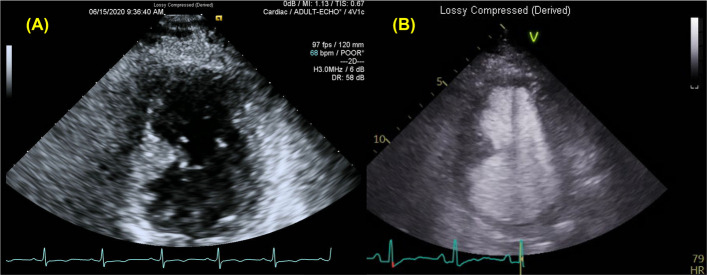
Contrast echocardiography. A Transthoracic echocardiography revealing thickening of the mid-inferoseptal wall, while the apical myocardium not being clearly visualized. B Following contrast injection, the apical myocardium becomes well visualized
